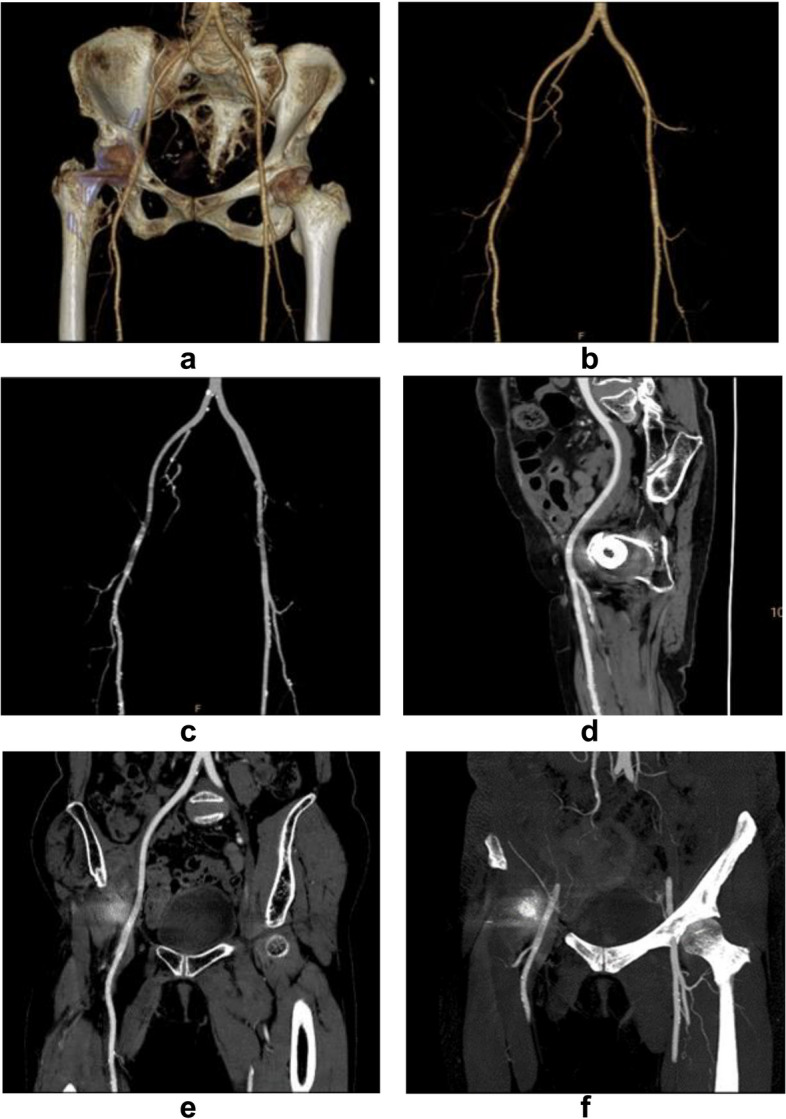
A series of post-processing CTA images of the same patient. 3D volume rendering (VR) (a), 3D VR without bone (b), maximum intensity projection (MIP) without bone (c), curved plannar reconstruction (CPR) (d, e), and slab MIP (f) images of the same patient on VMI_MAR_ at 80 keV. Images show most artifacts are removed and the vessel is slightly affected with acceptable vascular attenuation and sharp vascular contour
